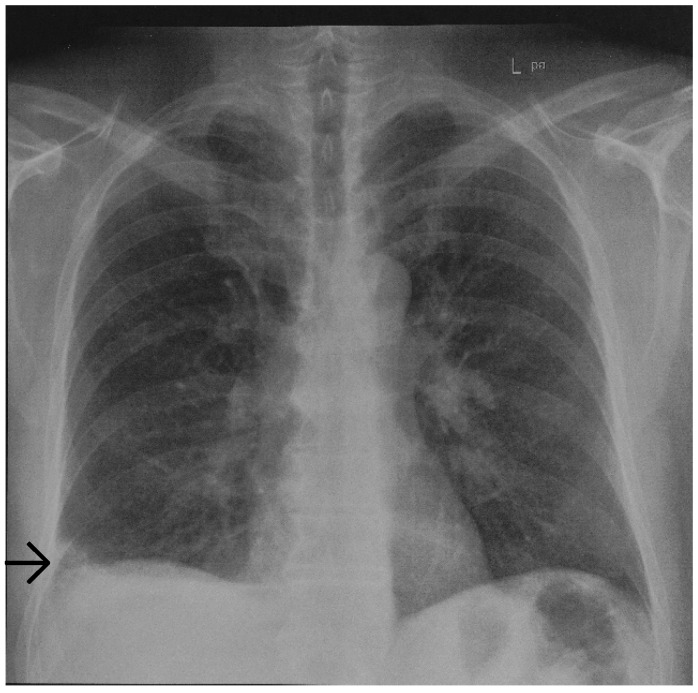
Posterior-anterior view of a chest radiograph demonstrating a basal wedge-shaped consolidation indicative of a Hampton’s hump (arrow) in the right lower lobe.
